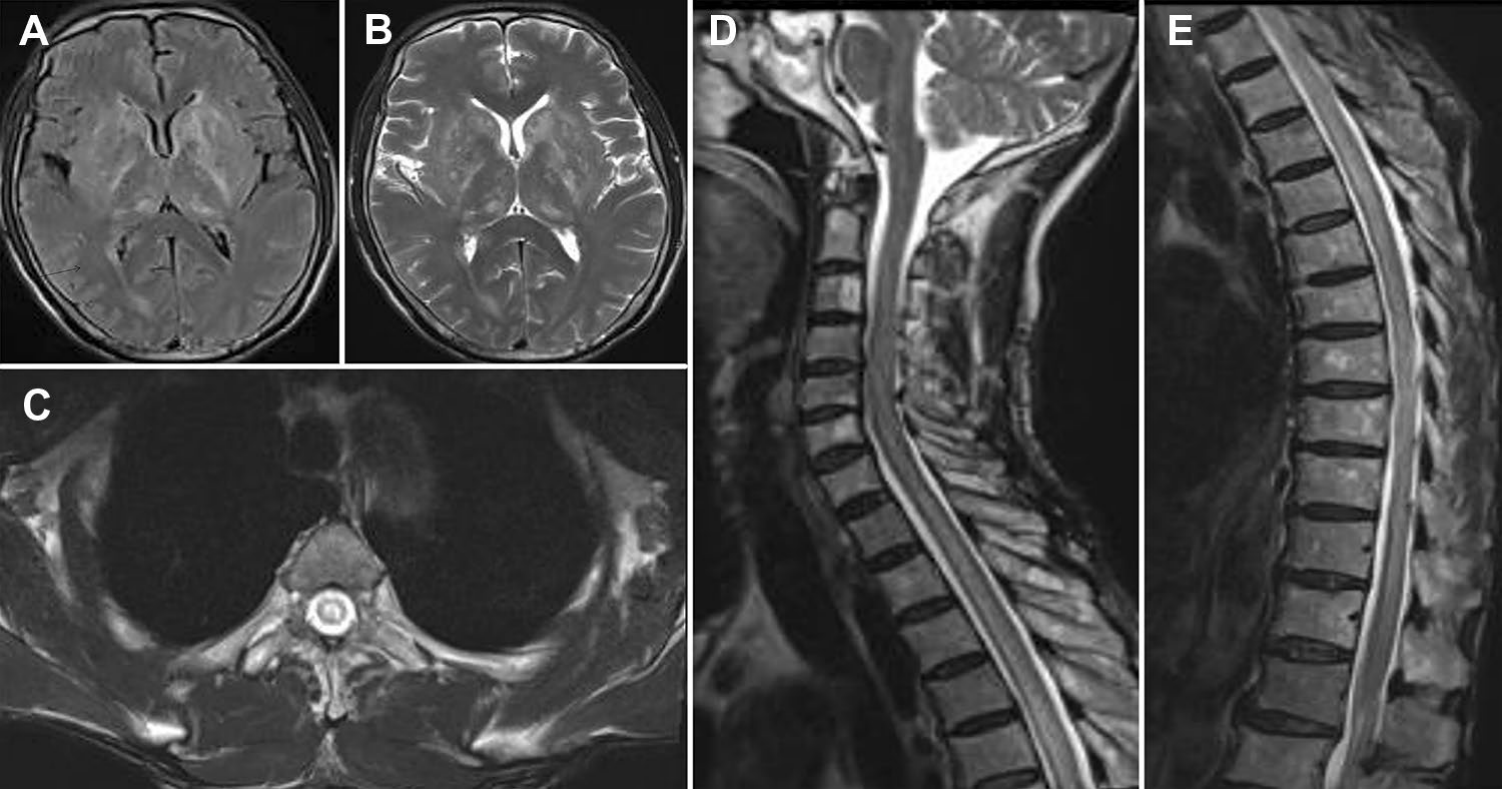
Brain MRI showing T2 patchy signal abnormalities in the bilateral basal ganglia, thalamus, and right corpus callosum, with the Flair image revealing high signals. (A, B). A slightly thickened spinal cord in the cervicothoracic segment, in which the slightly long T2 signals could be seen (C, D). These signals were mainly in the central part of the spinal cord (E)
